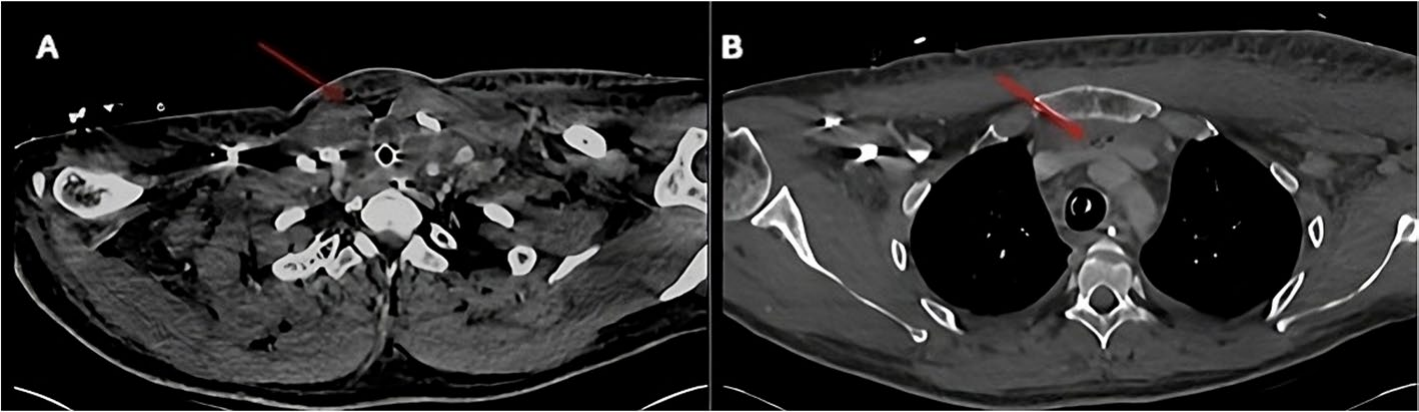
Post-operative CT scan on day 5 (axial section) demonstrates collections in the surgical bed (red arrow) (A). Fluid and gas locules within the retrosternal space (red arrow – panel B). Collection extending into the superior mediastinum (B)
